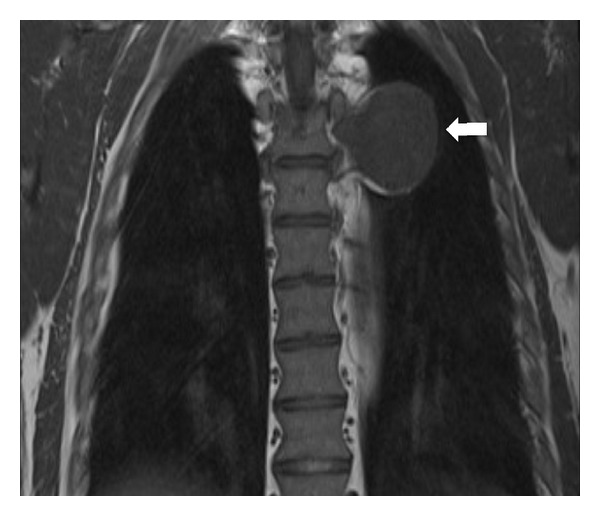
Chest MRI (coronal T1-weighted turbospin echo sequence) in a 50-year-old male patient with Castleman disease. The left paraspinal pleural-based mass is slightly hyperintense to skeletal muscle on T1-weighted sequences (arrow).
